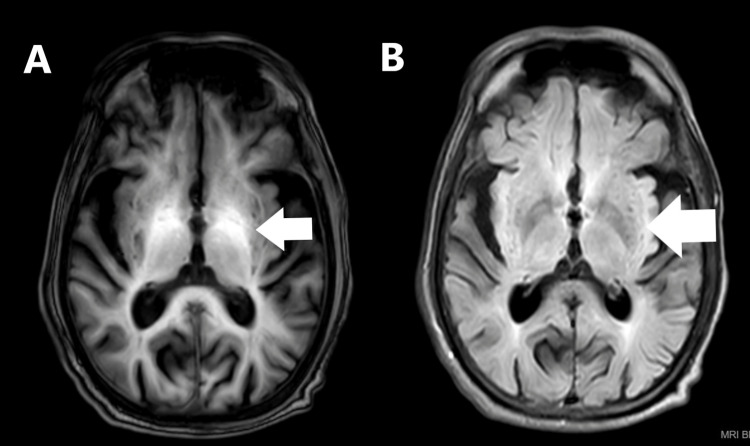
MRI brain axial T1 image (A) at the level of the gangliocapsular region shows symmetrical hyperintensity in bilateral globus pallidus (arrow) and corresponding hypointensity of the bilateral globus pallidus in T2/fluid-attenuated inversion recovery image (B).
